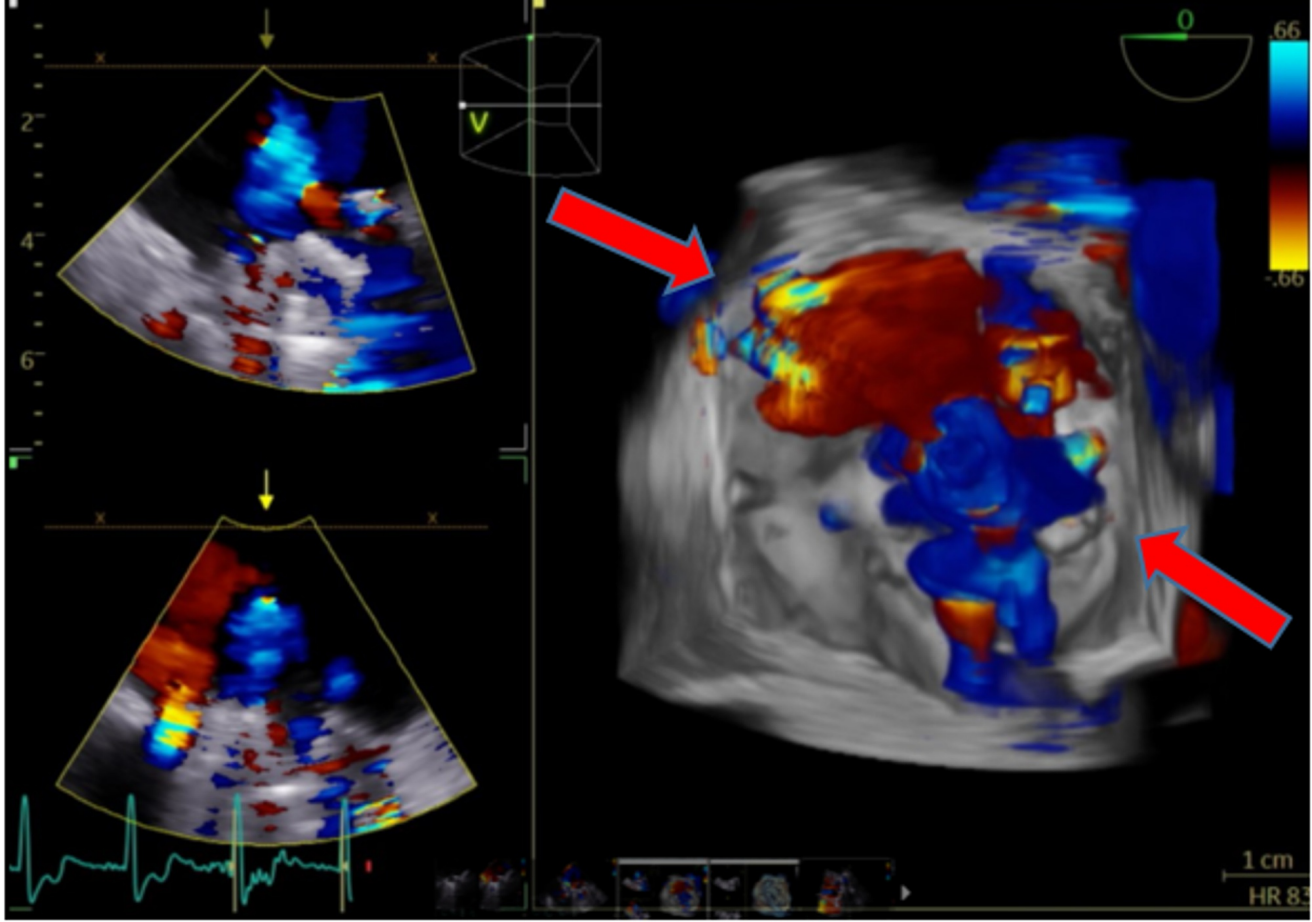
3D transesophageal echocardiogram of mitral valve showing multiple paravalvular regurgitant jets on color Doppler (red arrow)
